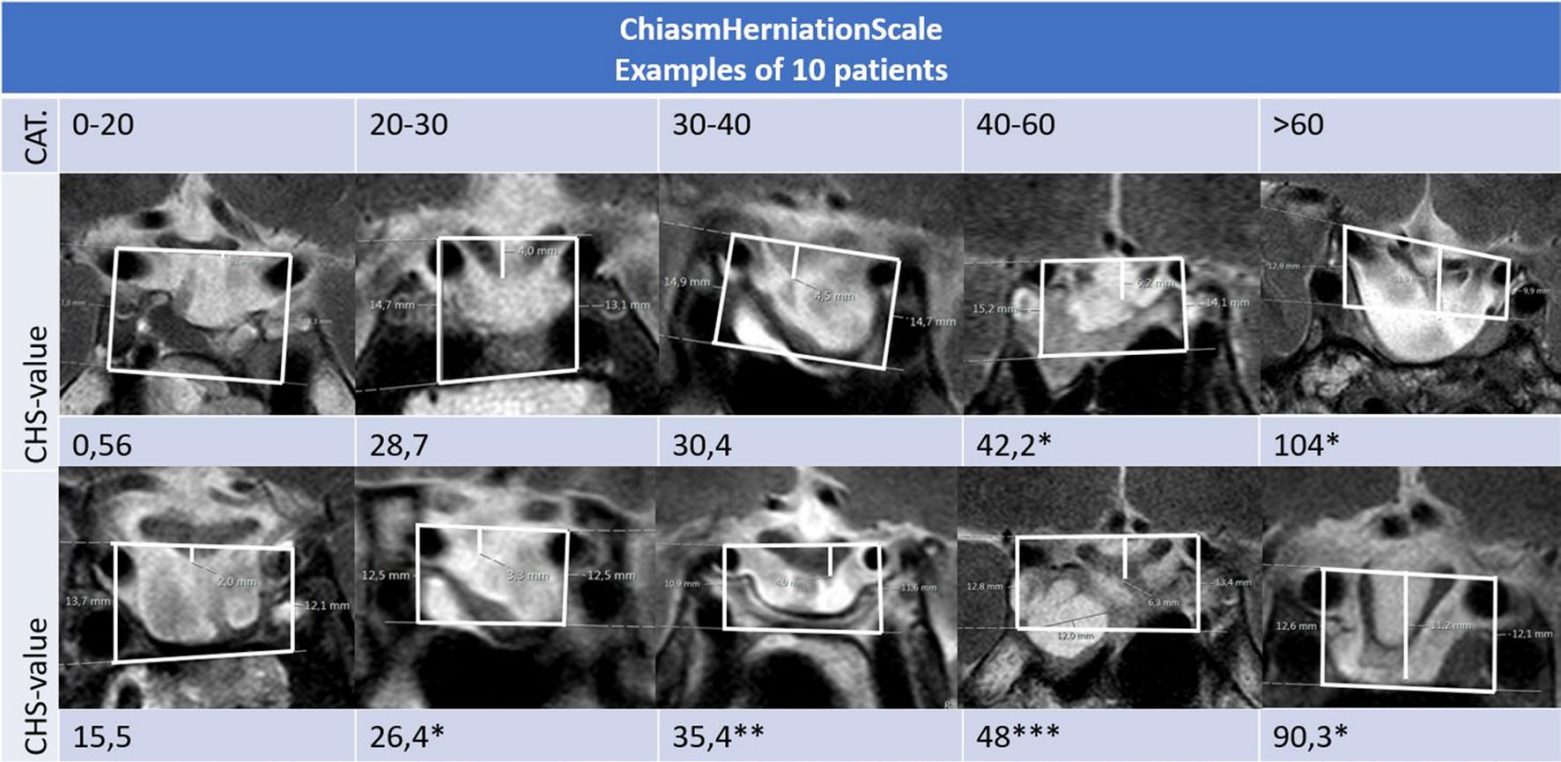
MRI examples of the five categories on the CHS for ten different patients. For each of the five CHS categories two MRI’s are demonstrated with the exact CHS-value below. All MRI’s are obtained from different patients and include the same lines as used in Fig. 1 for calculation of the CHS-value. *With extrapolation of the optic chiasm. With extrapolation of the line under lower carotid arteries. *In the left lower corner, a tumour residual is visible. There is no compression on the optic chiasm. *CAT* category
